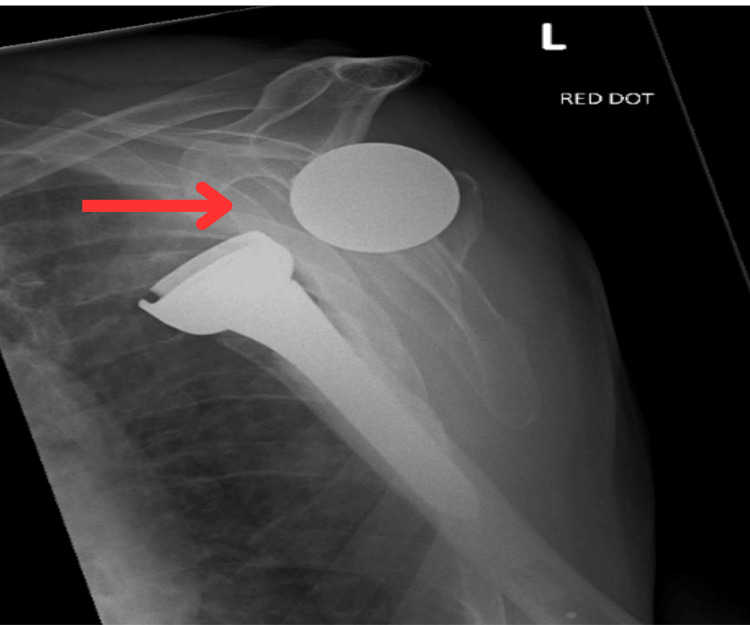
Radiograph confirming the first anterior dislocation of the reverse total shoulder replacement
